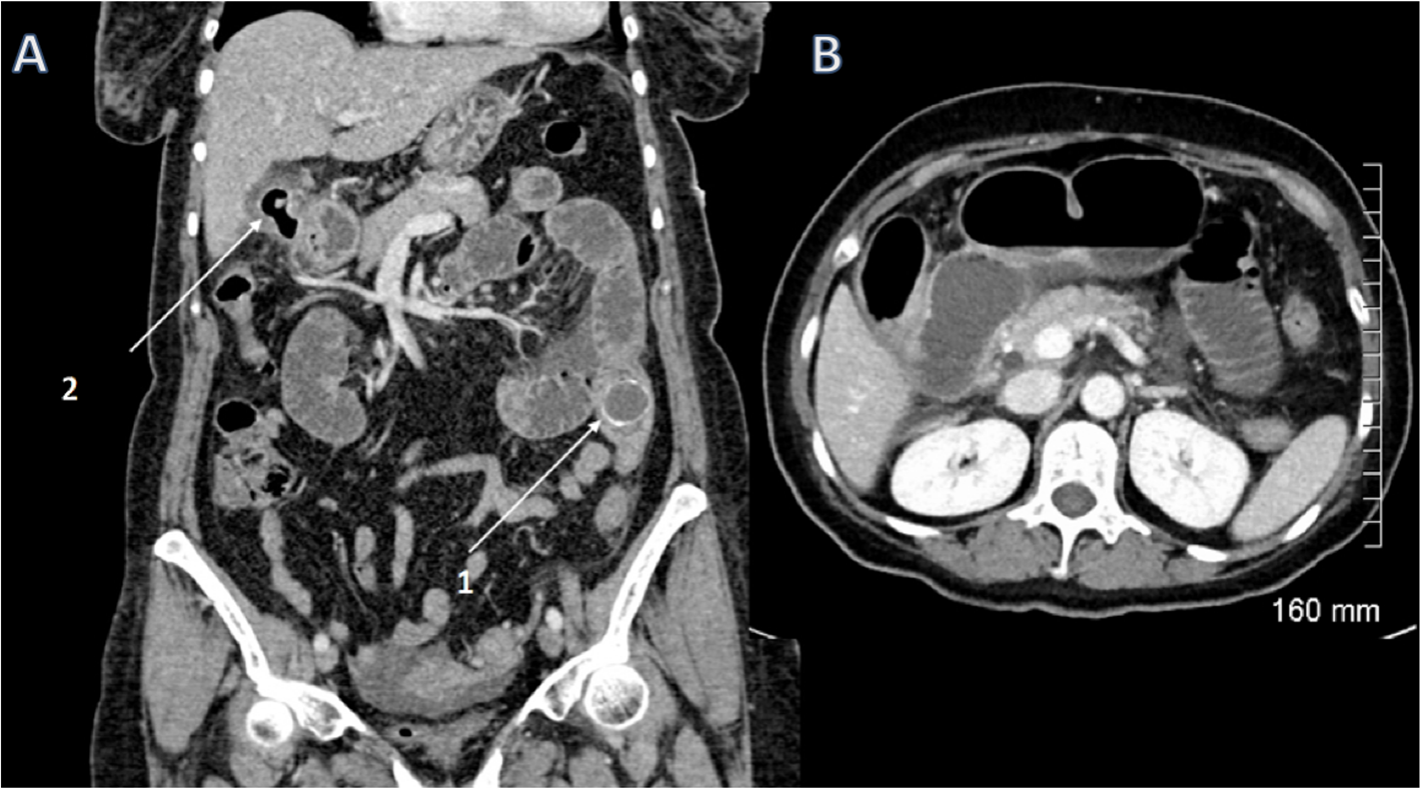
Coronal (a) and axial (b) contrast-enhanced CT of the abdomen demonstrating multiple dilated loops of small bowel. There is a 3-cm peripherally hyperattenuating obstructing gallstone in the left flank (arrow 1). There is an extensive inflammatory process in the gallbladder bed with air in the gallbladder (arrow 2) consistent with a cholecystoenteric fistula. Appearances are consistent with a bowel obstruction secondary to a gallstone ileus
